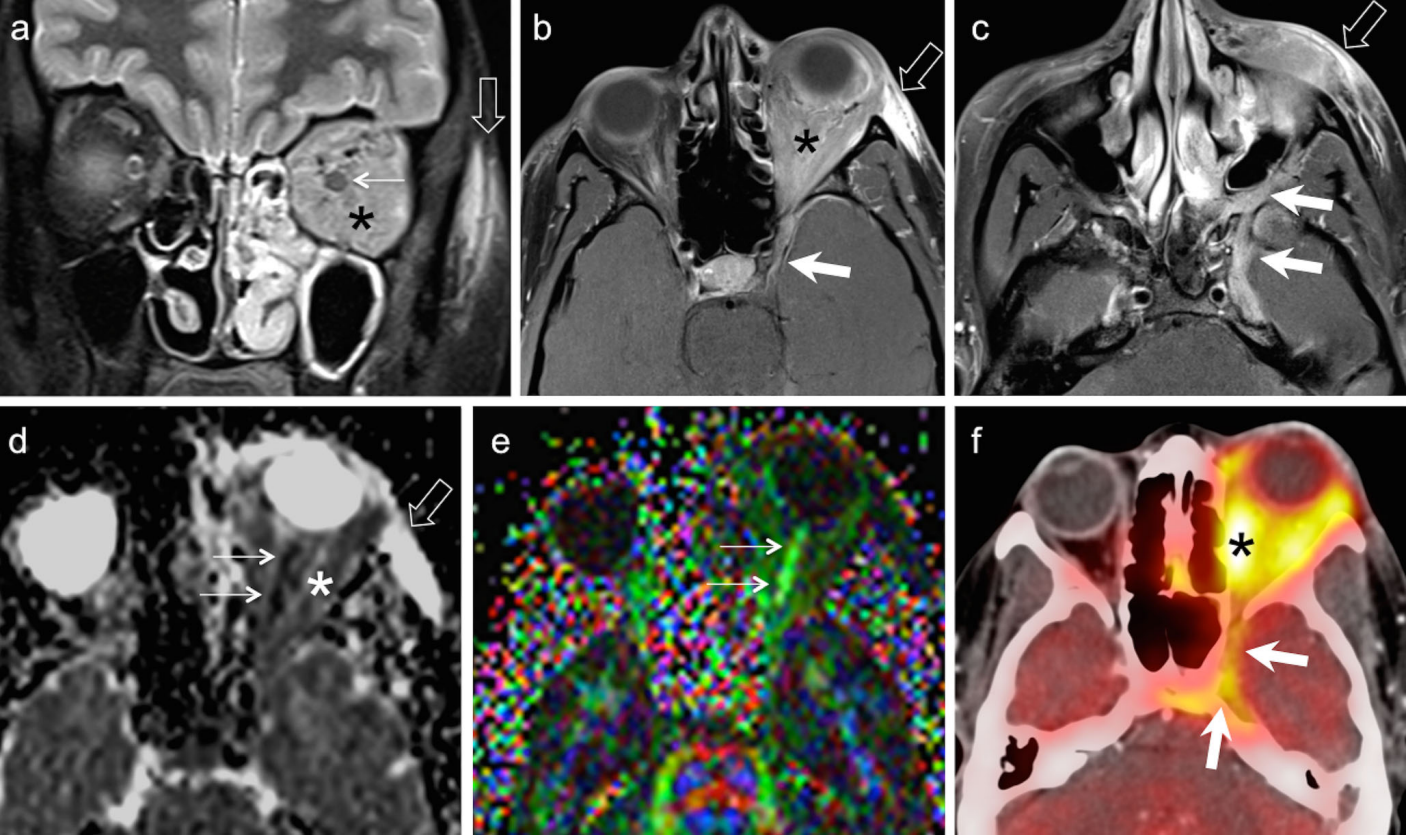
35-year-old female patient with painful proptosis, loss of vision, and subcutaneous facial swelling. Biopsy of the face performed in an outside institution suggested inflammatory pseudotumour. a. Coronal STIR image shows an ill-defined, moderately hyperintense lesion involving the entire left orbit (asterisk) and encasing the optic nerve (thin arrow). The subcutaneous hyperintense, poorly defined area on the left (hollow arrow) corresponds to the biopsied region. b. Axial contrast-enhanced FS T1W MR image of the same patient as in a. The left orbital lesion shows diffuse post-contrast enhancement (asterisk). Enhancing soft tissue is seen extending along the left superior orbital fissure into the left cavernous sinus (arrow) and the dura along the left greater wing of sphenoid. c. Axial contrast-enhanced FS T1W image at the level of the maxillary sinus demonstrates perineural spread along the pterygopalatine fossa and maxillary nerve (arrows). Hollow arrows in b and c point at extra-orbital involement. d. ADC map reveals restricted diffusion of the orbital lesion with very low ADC values (ADC = 0.7 × 10 ^−3^ mm^2^/s) suggesting lymphoma. The optic nerve shows even lower ADC values (thin arrows) due to compression and ischemia. e. Colour coded DTI map shows major reduction of FA values in the left optic nerve (thin arrows). FA values were 0.4–0.5 on the left and 0.56–0.58 on the right. f. FDG PET/CT demonstrates high tracer uptake in the orbit (asterisk), along the superior orbital fissure and in the cavernous sinus and sphenoid (arrows) confirming findings revealed in b. SUVmean = 10, max = 16. Other hypermetabolic lesions were found in the neck nodes, mediastinum, and abdomen. Biopsy of orbital contents revealed NHL
